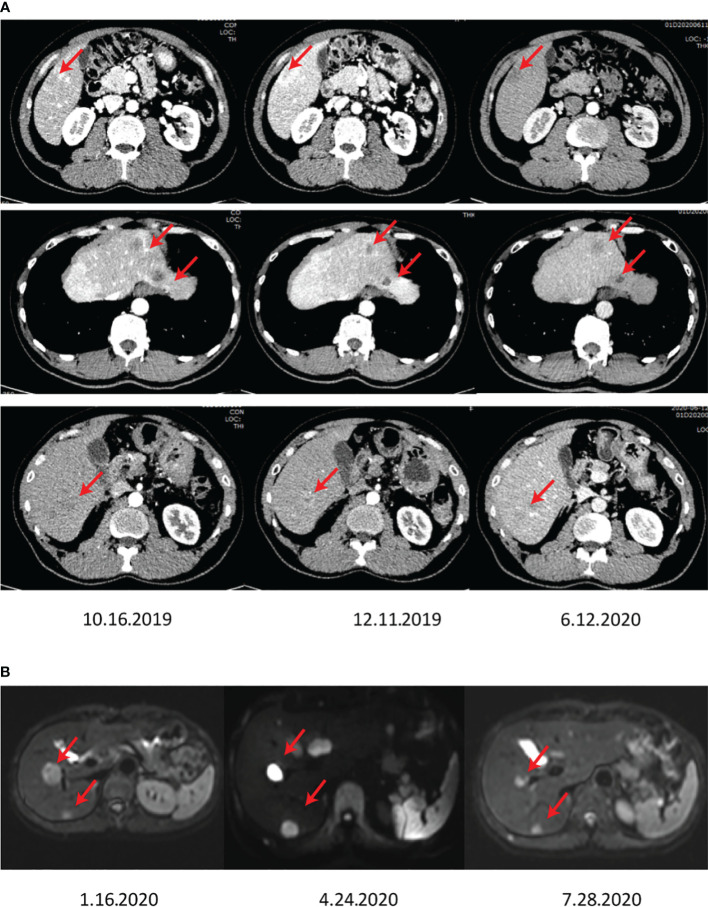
The images show the abdominal CT (A) and MRI (B) after surgery, showing growing lesions in the residual liver.
